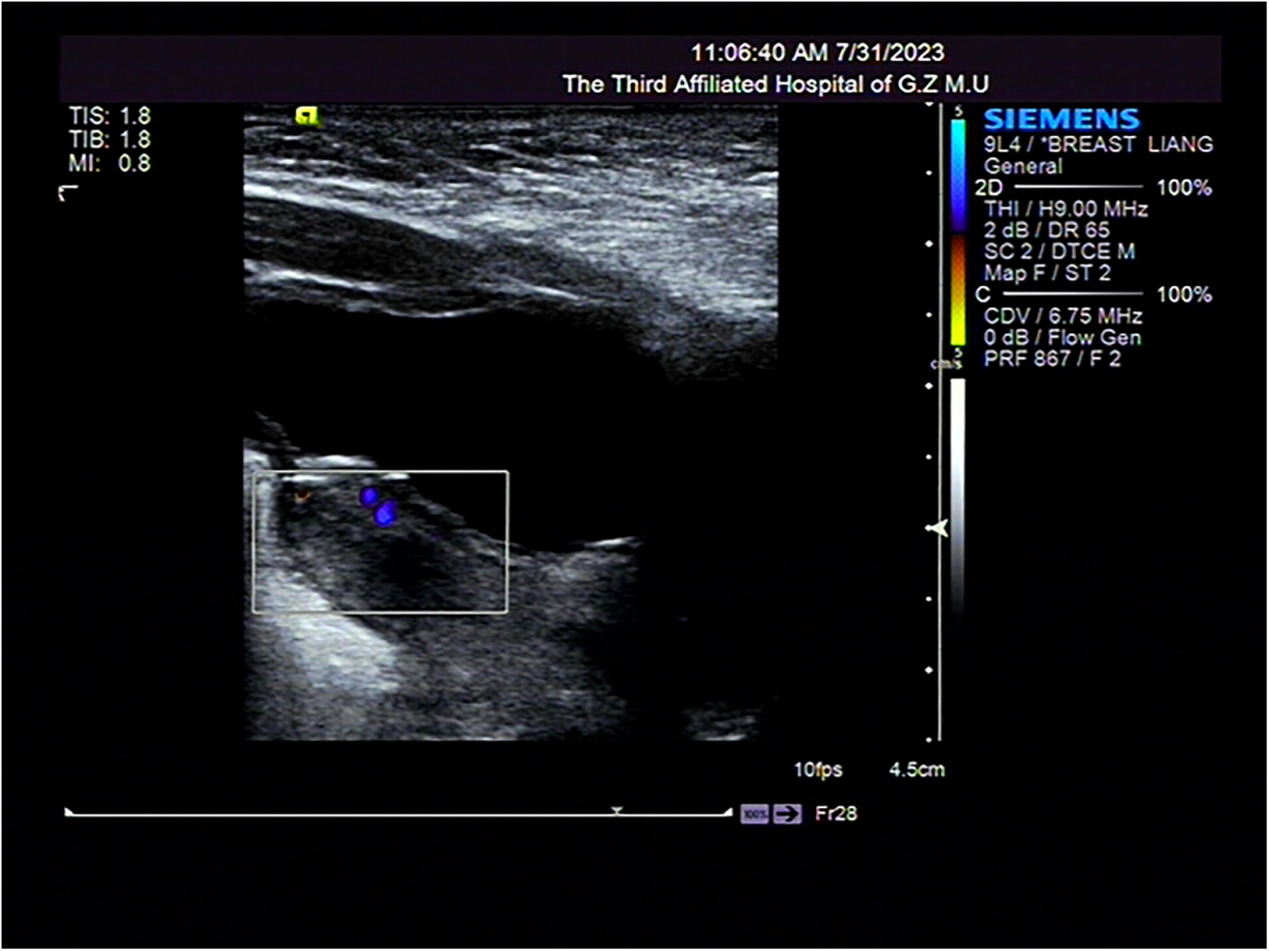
Pelvic and abdominal ultrassonography imaging of the first-born twin.
